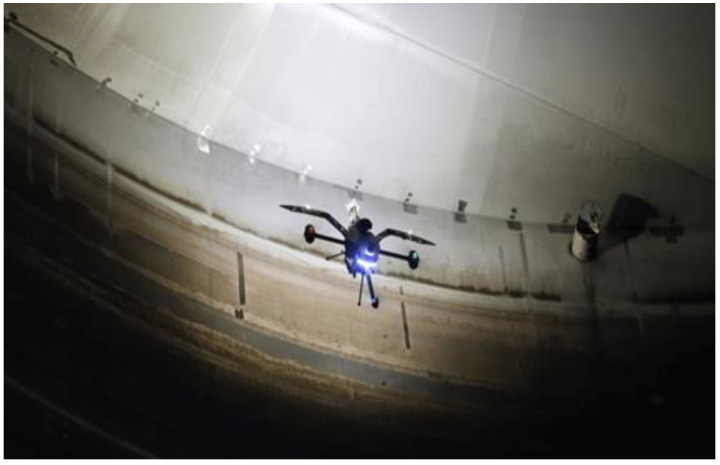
Drone for ultrasonic wall thickness measurement.
